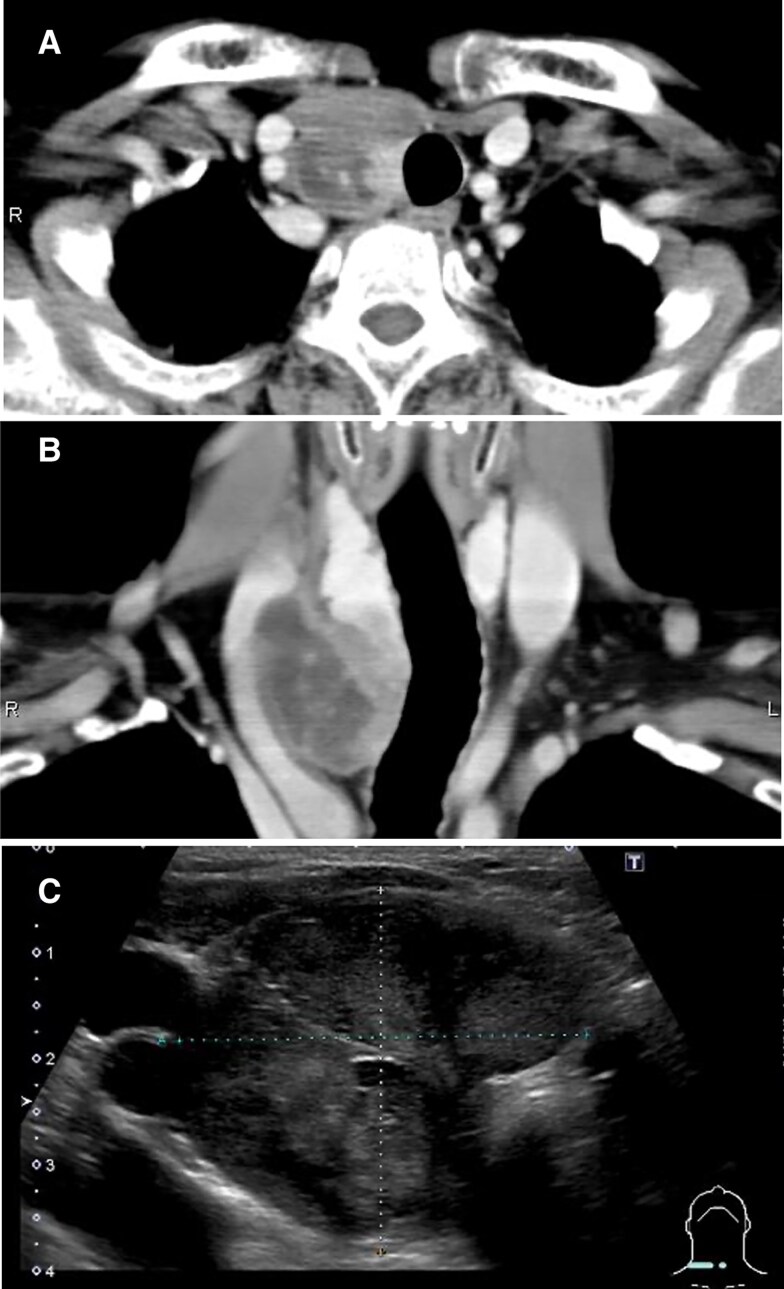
Cervical computed tomography (A, B) and ultrasonography (C) 7 months after presentation. Shrinkage of the cystic components is noted.
